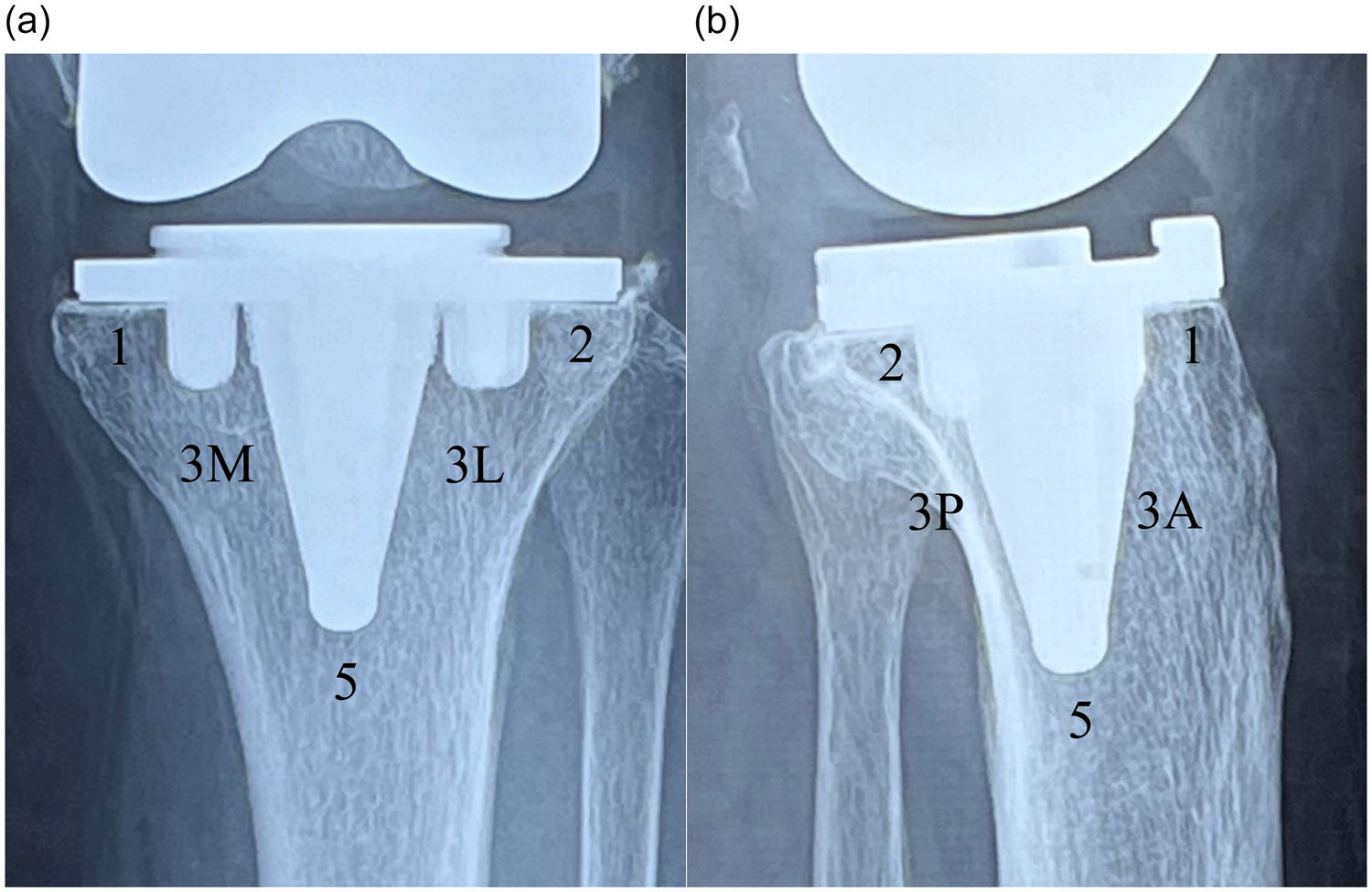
(a) Coronal radiographs of implants with zones for documentation of radiolucent lines. Zone 1: medial baseplate. Zone 2: lateral baseplate. Zone 3: central keel region. ‘M’ and ‘L’ are used to designate ‘medial’ and ‘lateral,’ respectively. Zone 5: the most inferior area of the keel. (b) Sagittal radiographs of implants with zones for documentation of radiolucent lines. Zone 1 of the tibia: anterior baseplate. Zone 2 of the tibia: posterior baseplate. Zone 3 of the tibial: central keel region. ‘A’ and ‘P’ are used to designate ‘anterior’ and ‘posterior,’ respectively. Zone 5 of the tibia: the most inferior area of the keel.
